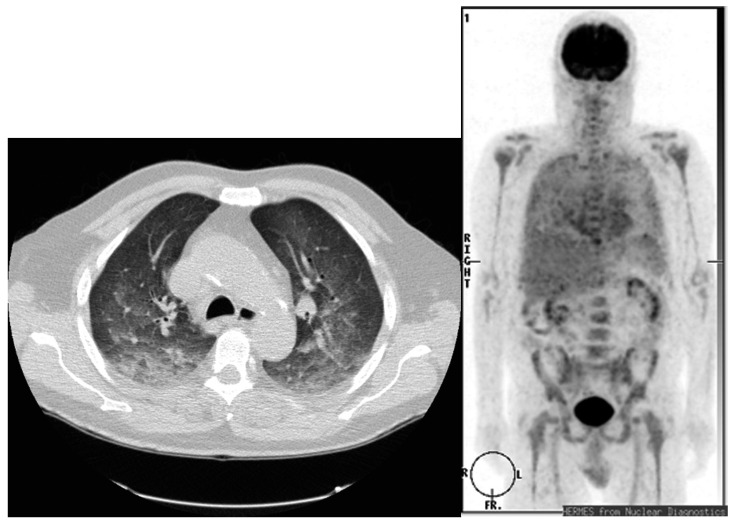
Pulmonary toxicity in a patient with chronic myelomonocytic leukemia treated with etoposide. A computer tomography (CT) scan (left panel) shows diffuse ground glass and reticular opacities in bilateral posterior pulmonary segments. A coronal ^18^F-fluorodeoxyglucose (FDG) positron emission tomography (PET) scan (right panel) demonstrates bilateral diffuse pulmonary uptake (Used with permission from [29]).
